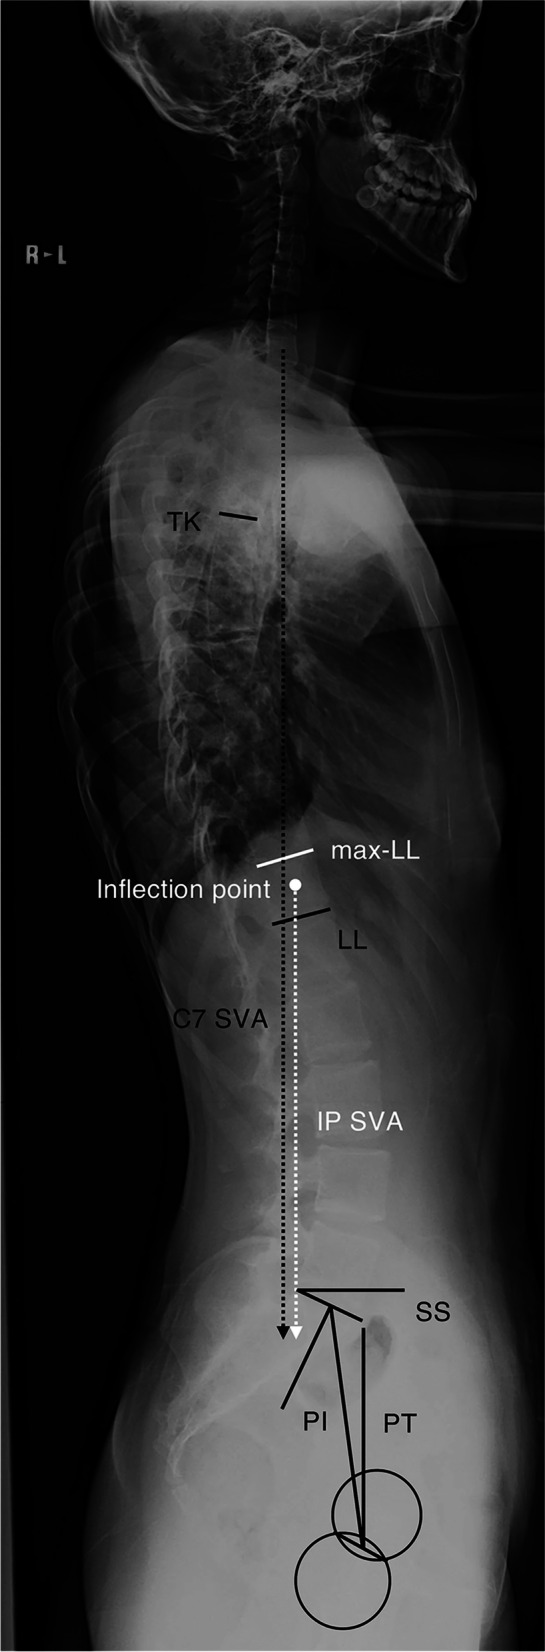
Radiographic parameters were measured on lateral images of the whole spine while the patients were standing. Max-LL: maximum lordotic Cobb angle from S1, IP: inflection point between thoracic kyphosis and lumbar lordosis, IP SVA: sagittal vertical axis of IP
